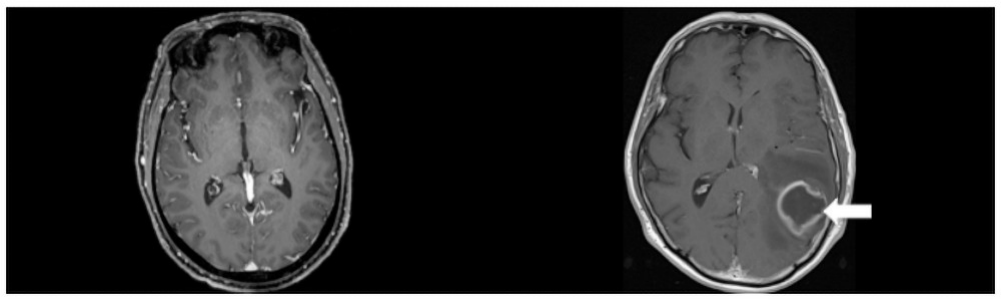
Exemplary stimuli used in our study, showing T1-weighted post-contrast MR images of a normal (left image) and a pathologic (right image) brain. The arrow indicates a glioblastoma. Note, the arrow was not shown to participants.
